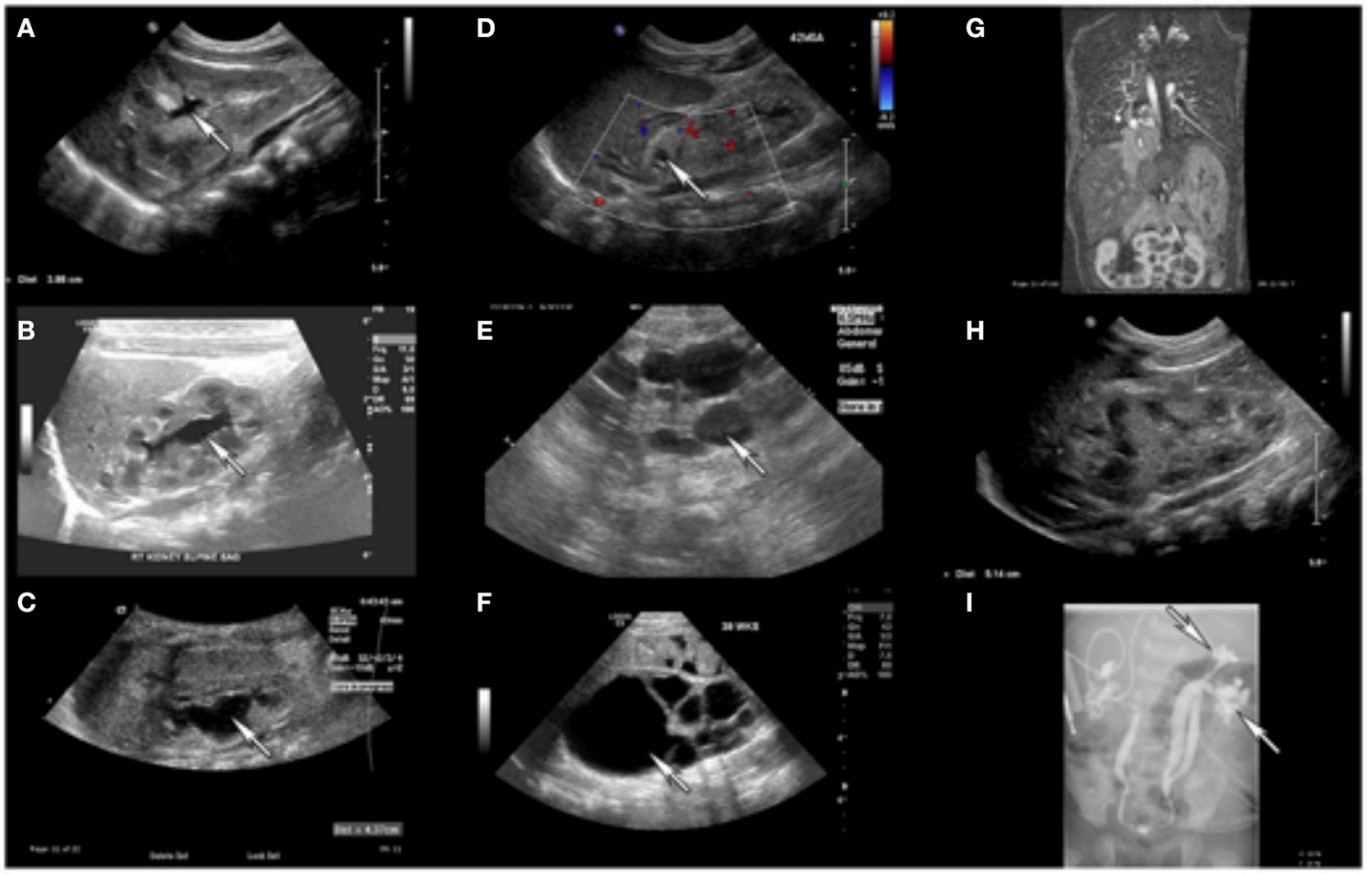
Kidney Defects Observed in Patients with CHD. Renal ultrasound of patients with congenital heart disease unveils a spectrum of renal abnormalities including intrarenal collecting system dilation (A–C), cystic-dysplastic abnormalities (D–F), horseshoe kidney (G), and duplicated collecting system depicted with renal ultrasound (H), and voiding cystourethrogram (I). Adapted from San Agustin et al. (10).
